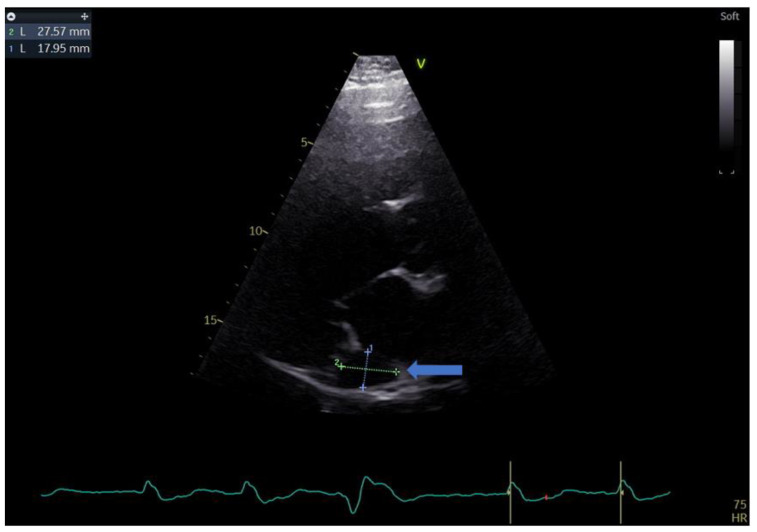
Parasternal long-axis view showing the measurements of the coronary sinus (blue arrow).
